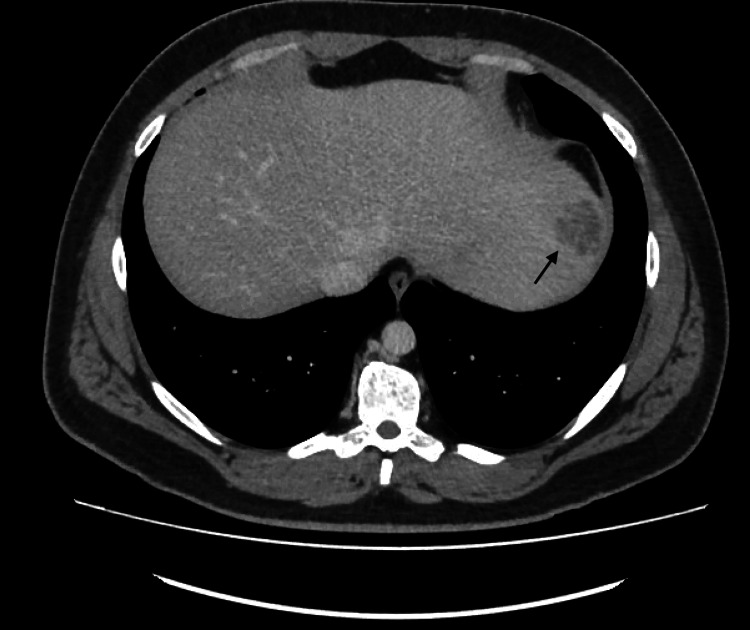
CT abdomen CT abdomen showing a lesion on the upper left lobe of the liver (as shown by the arrow)
